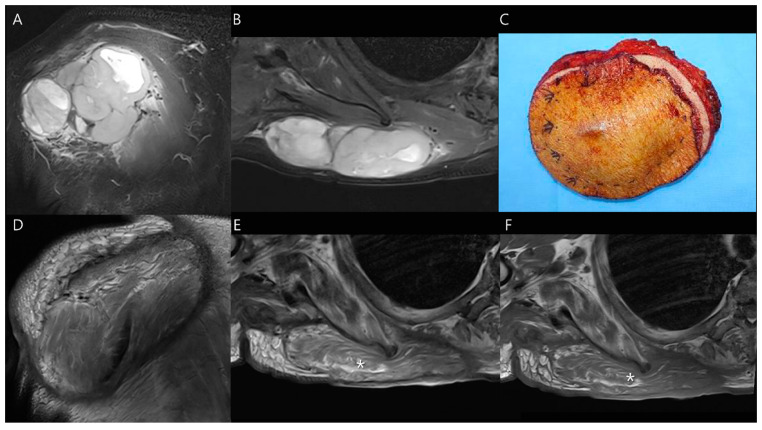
Wide excision for undifferentiated pleomorphic sarcoma on the posterior chest wall. (A,B) Preoperative coronal and axial T2-weighted fat-suppressed MR images show a 10 cm soft tissue mass. (C) The tumor was removed through a wide excision, and the resultant defect was covered with a free latissimus dorsi flap. (D,E) Postoperative coronal and axial T2-weighted MR images after 6 months demonstrate successful coverage of the defect by the flap, confirming proper flap integration with surrounding tissues. Note fatty infiltration within the flap area (asterisk in E). (F) Axial T1-weighted MR image shows fatty infiltration within the flap area (asterisk).
